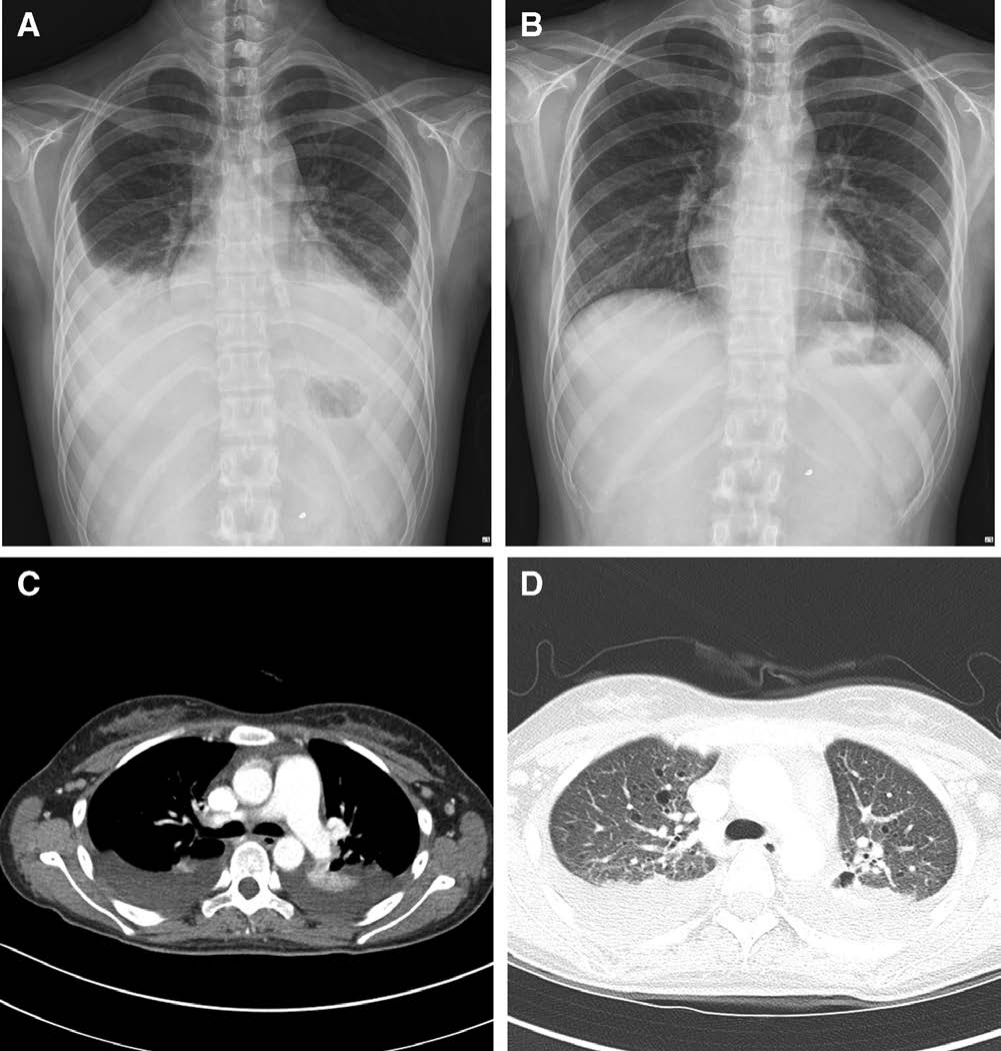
Chest images. (A) Chest X-ray showing bilateral pleural effusion after left renal selective TE. (B) Following treatment with diuretics and antibiotics, the patient’s chest X-ray findings markedly improved. (C) The mediastinal setting of the chest CT shows pleural effusion in both lungs. (D) The lung setting of the chest CT demonstrates multiple cystic lesions of the lung parenchyma. TE = transcatheter embolization, CT = computed tomography.
